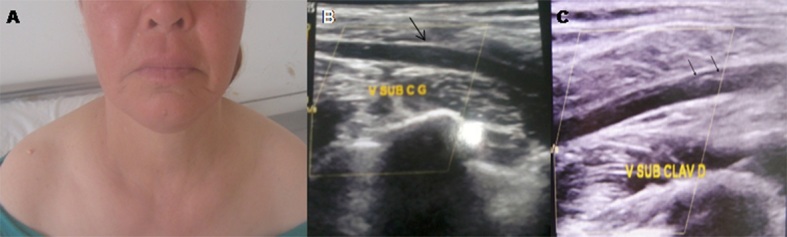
(A) comblement des creux sus claviculaires. Echographie Doppler des membres supérieurs: absence de flux au niveau des veines subclavières (B) gauche, et (C) droite; qui sont dilatées avec un contenu échogène (fleches)
